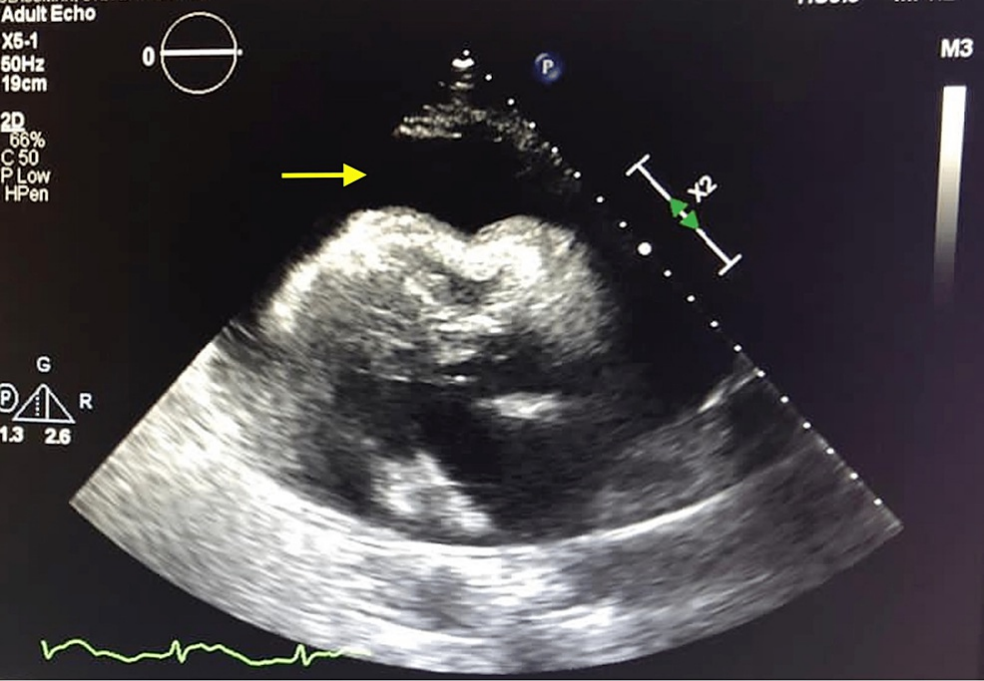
2D transthoracic echocardiogram on admission showing large pericardial effusion with diastolic collapse of right ventricle consistent with tamponade physiology
